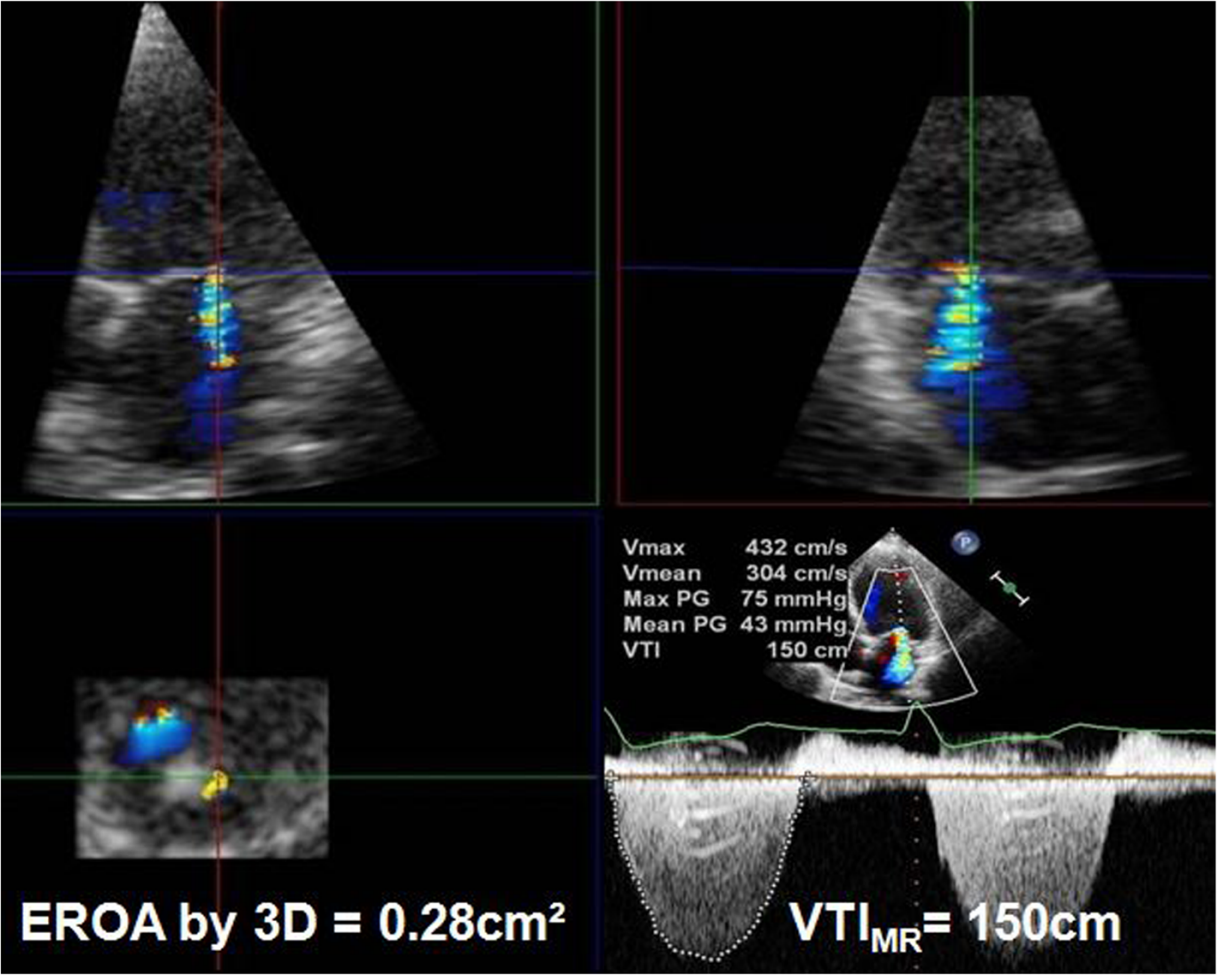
MRvol calculated as EROA by RT3DE multiplied by the VTI_MR_. The 3D color Doppler data was manually cropped by the cross-sectional plane perpendicularly to the regurgitant jet direction up to the smallest cross-sectional area of the regurgitant jet. The EROA was determined using manual planimetry of the color Doppler flow signal from an en face view. Example of a MR patient: EROA = 0.28 cm^2^, VTI_MR_ = 150 cm, MRvol = 0.28 × 150 = 42 mL
